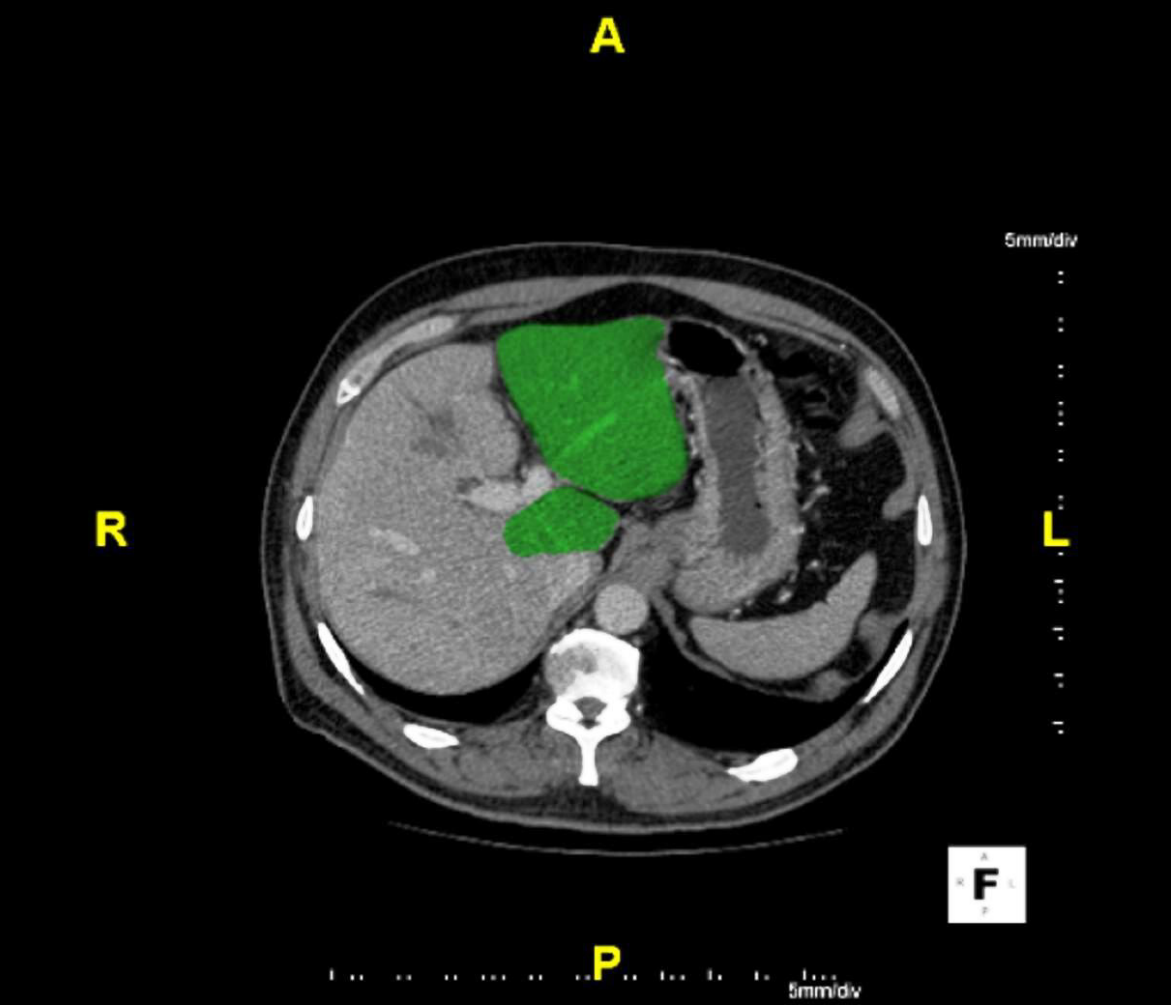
CLM after PVE for Small FLR Prior to Hepatic Resection. Patient(as in Figure 1) with metastatic rectal carcinoma and right hepatic metastasis who underwent right PVE(6/10/09) with followup CT(7/13/09) with demonstrable growth of the FLR.
